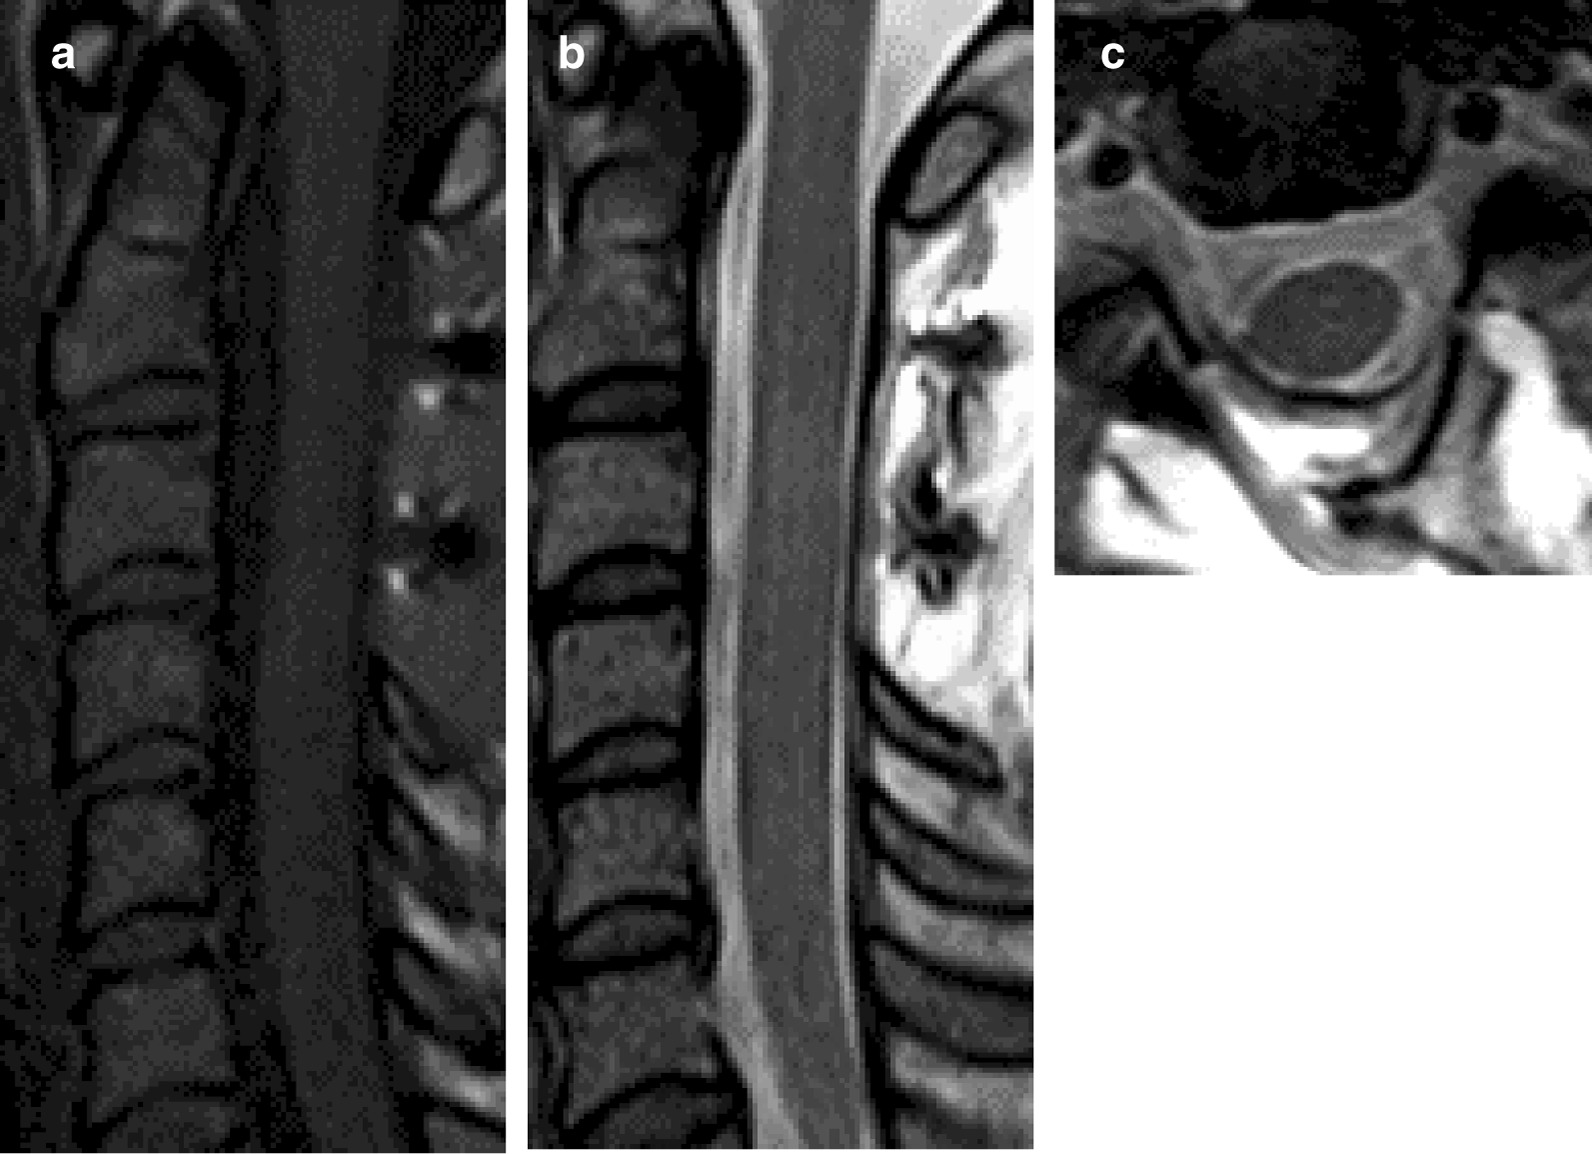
Postoperative magnetic resonance imaging (MRI) of the cervical spine. a Mid-sagittal T1-weighted image; b mid-sagittal T2-weighted image; and c axial T2-weighted image at the C3 vertebra
